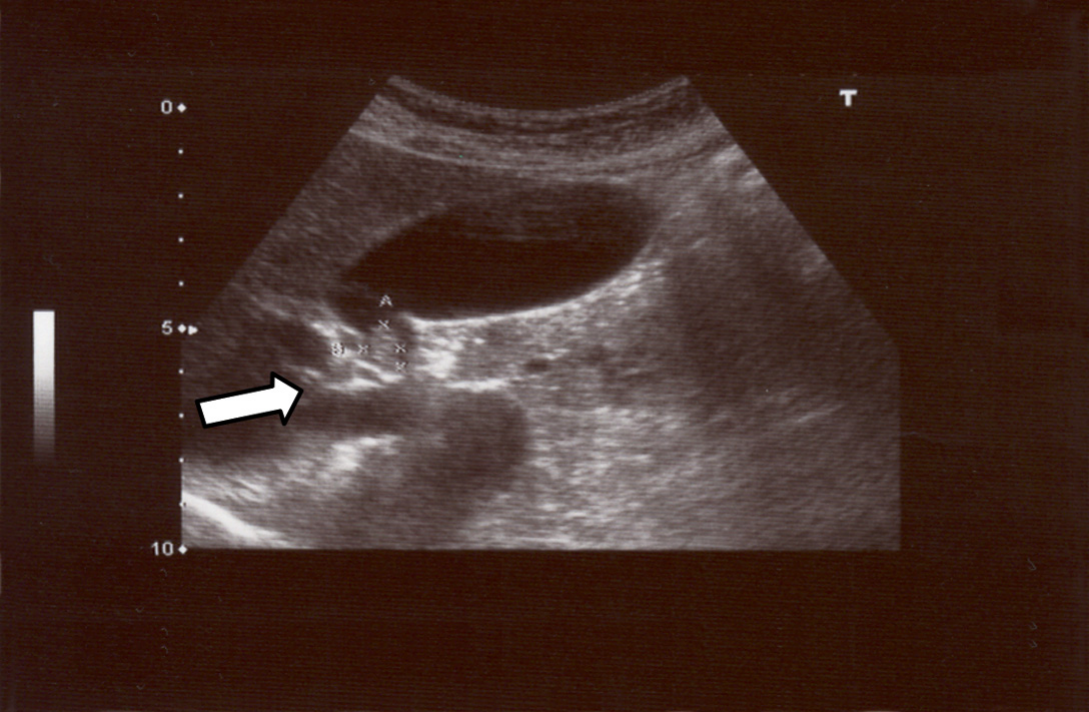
Abdominal ultrasound image of a polypoid mass between the neck and body of a gallbladder.
